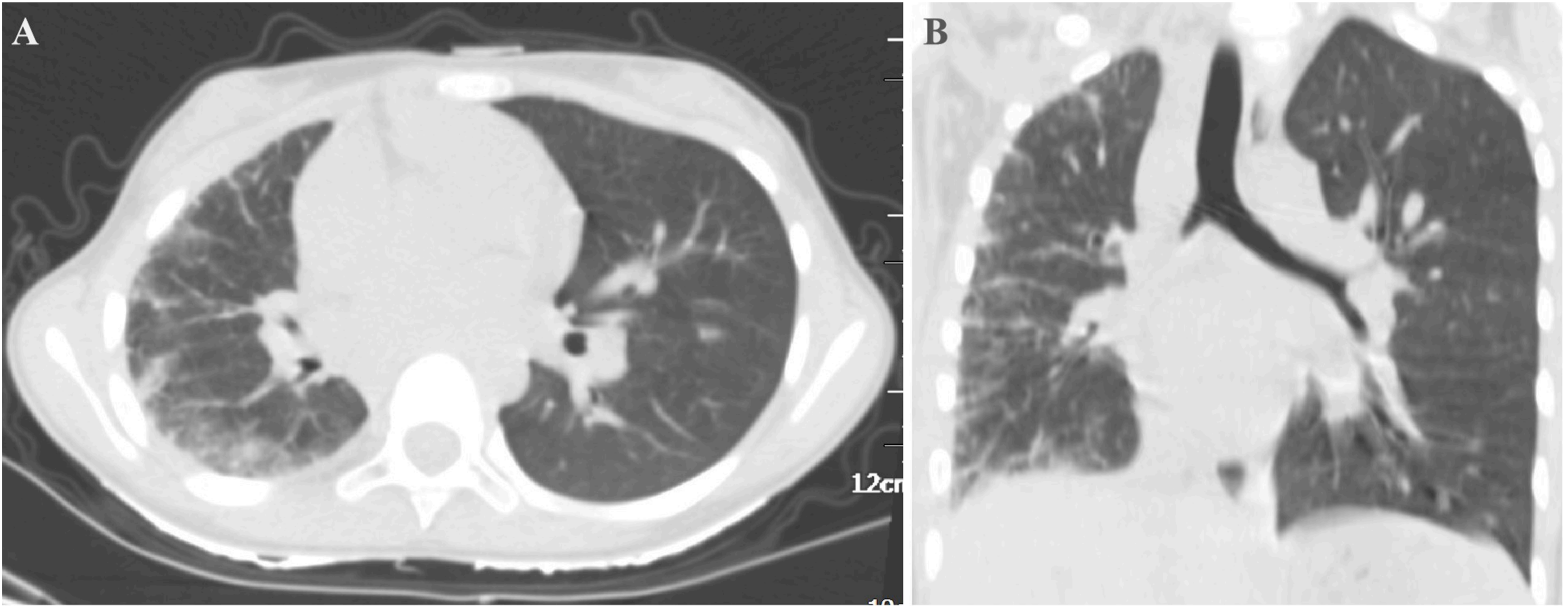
A) Chest CT scan (transverse view) and B) chest CT scan (coronal view) of Case No. 81 after 5 years and 4 months of follow-up, showing hypoplasia of the right lung, and increased interstitial lesions with irregular pleural thickening. CT = computed tomography.
